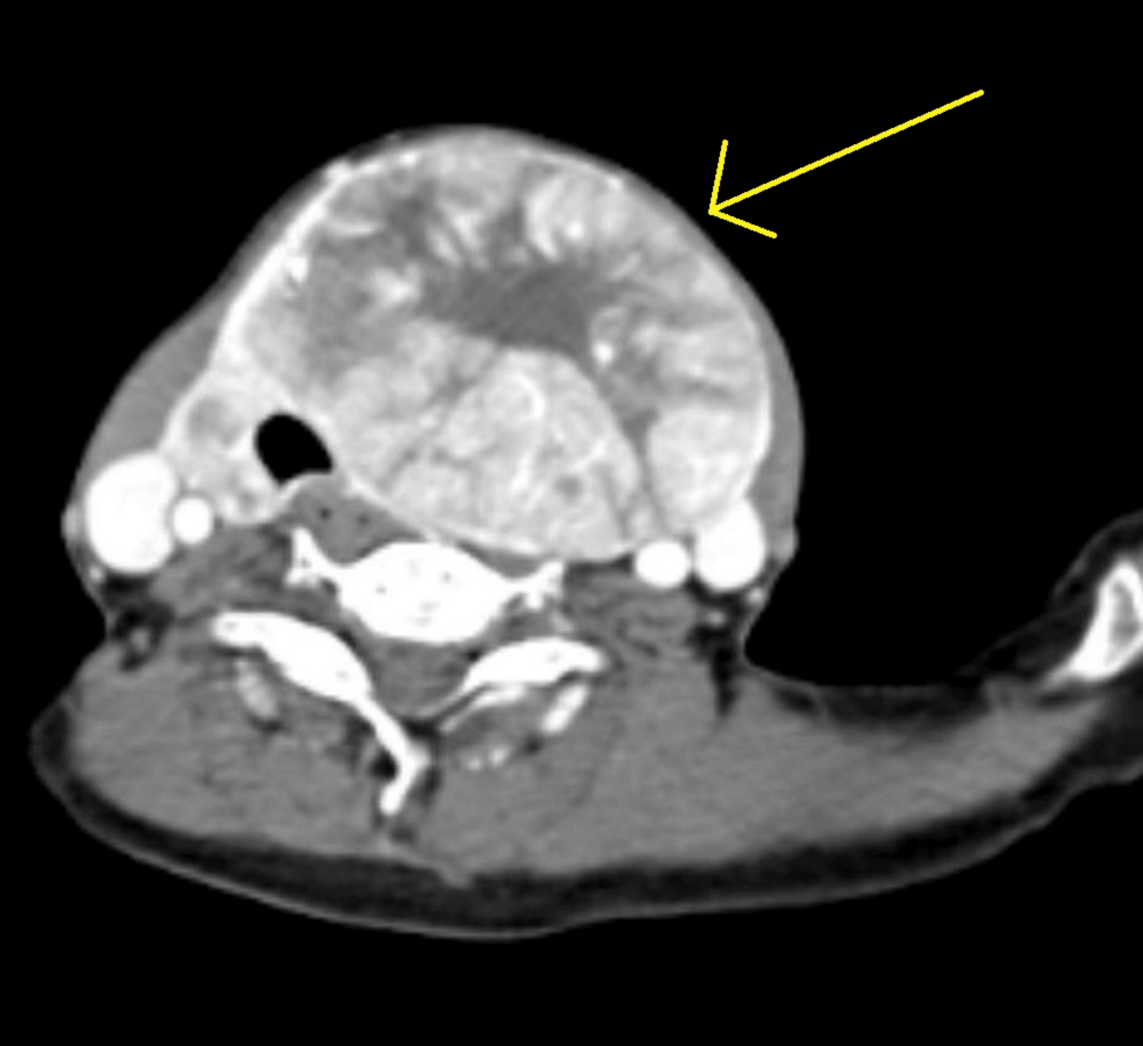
CECT showing the thyroid nodule (axial view) CECT: contrast-enhanced computed tomography
